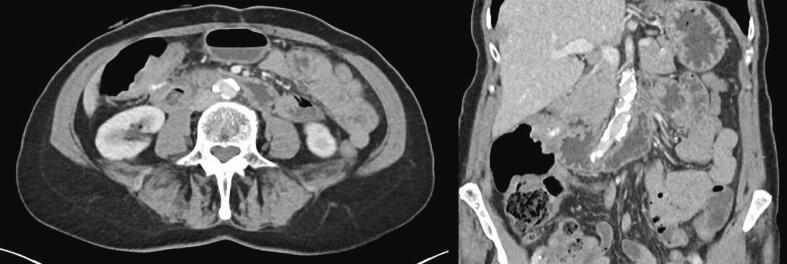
The CT scan following neoadjuvant treatment that demonstrates a remarkable response compared to the CT scan prior to this treatment.
